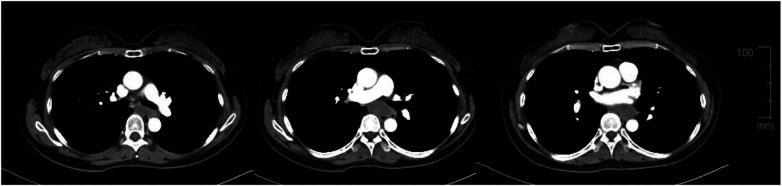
Preoperative contrast-enhanced CT showing the anatomical relationship of the leiomyoma. The image demonstrates that the esophageal leiomyoma is closely adjacent to the heart and major vessels, highlighting the high risk of major intraoperative bleeding and potential technical challenges for resection.
